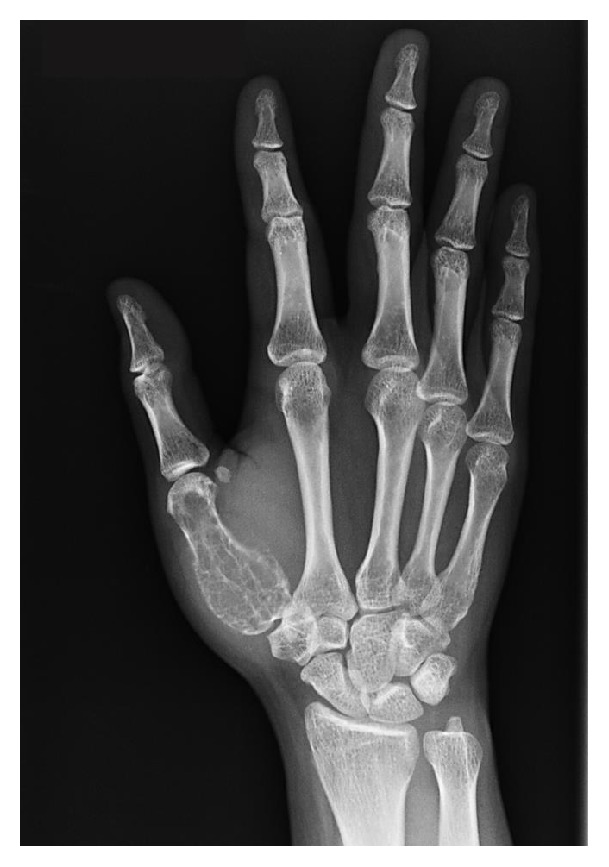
Plain radiograph showing expansile osteolytic lesion involving the entire length of the first metacarpal.
